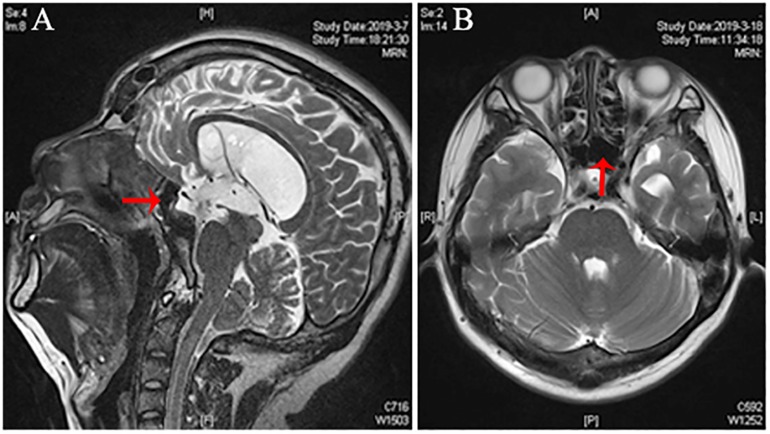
Post-ETV MRI of the patient with CSF rhinorrhea. (A,B) Post-ETV sagittal and axial T2-weighted MRI demonstrating CSF flow signal disappeared significantly compared to the preoperative image in the left sphenoidal sinus (arrow).
